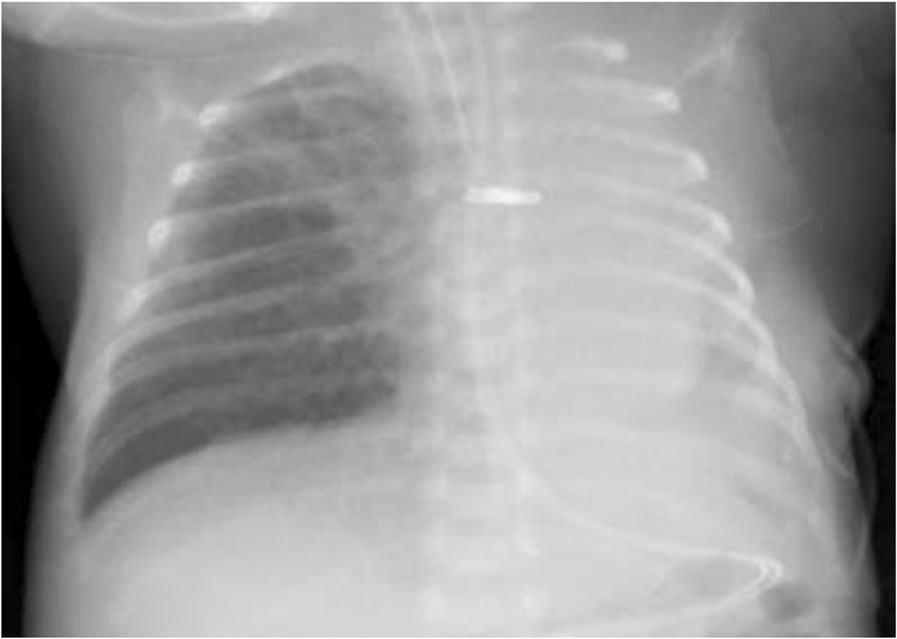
Chest X-ray after PDA ligation showed completely collapsed left lung
